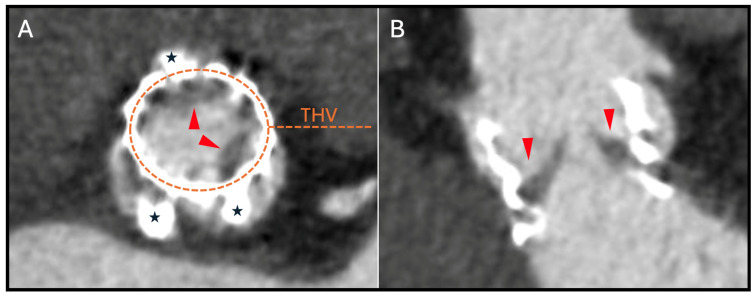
Example of hypoattenuated leaflet thickening in a balloon-expandable device. CT appearance of a THV on CT: (A) short axis and (B) double oblique transverse plane on a heavily calcified aortic valve (aortic calcification: black stars) with subclinical leaflet thrombosis (red arrowheads), characterized by hypoattenuated leaflet thickening (grade II). CT: computed tomography; THV: transcatheter heart valve.
